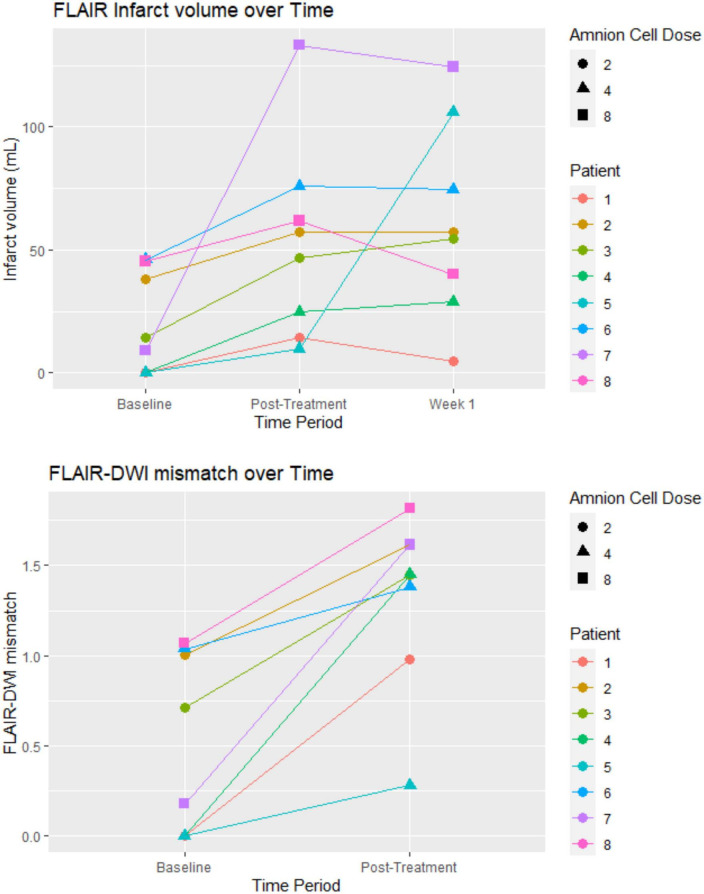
Infarct volume and FLAIR-DWI mismatch: the post-treatment was on the day that the hAEC infusion was completed. For patients receiving a dose of 8 × 10^6^ cells/kg the MRI was on day 2. The unit of hAEC dose was 10^6^ cells/kg. FLAIR, fluid attenuated inversion recovery sequence; DWI, diffusion weighted imaging.
